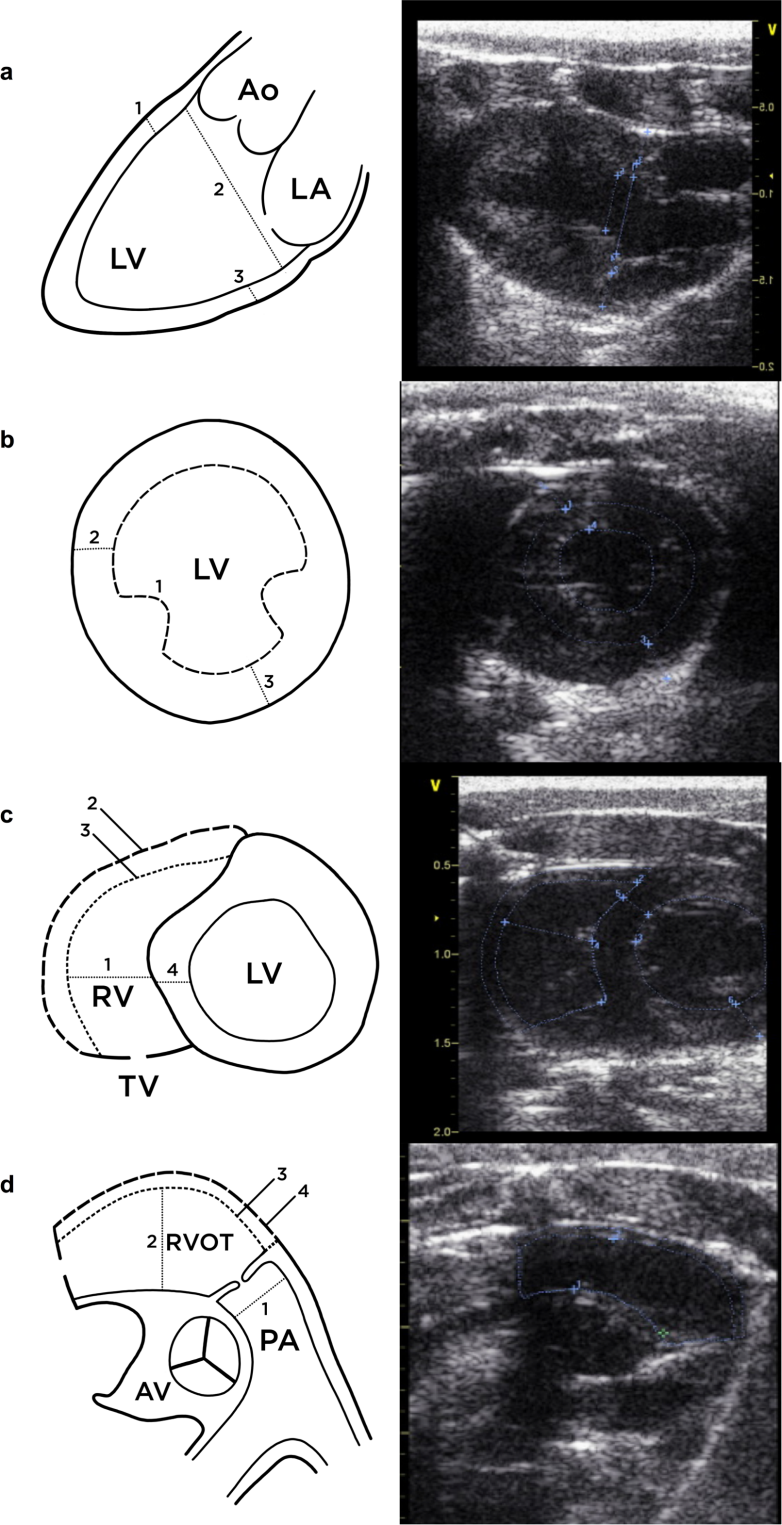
Echocardiographic views used to assess cardiac function. a. Parasternal long-axis (PLAX) view of LV (1. Interventricular septum IVS, 2 End-diastolic dimension LVIDD, 3. Posterior wall dimension PWD). b. Mid-ventricular SAX papillary view (1. LV end-diastolic chamber area LVEDA, 2. IVS, 3. PW). c. Mid-right ventricular (MRV) view, modified from Scherrer-Crosby[7], (1. R1 mid-chamber dimension, 2. Sub-epicardial chamber area RV_epi_, 3. Sub-endocardial chamber area RV_endo_, 4. IVS). d. Short axis (SAX) right ventricular outflow tract (RVOT) view (1. Main pulmonary artery diameter PAΦ, and locus for PWD measurement of ejection velocity time integral VTI 2. RVOT dimension R2, 3. Sub-epicardial RVOT chamber area RVOT_epi_, 4. Sub-endocardial RVOT chamber area RVOT_endo_).
